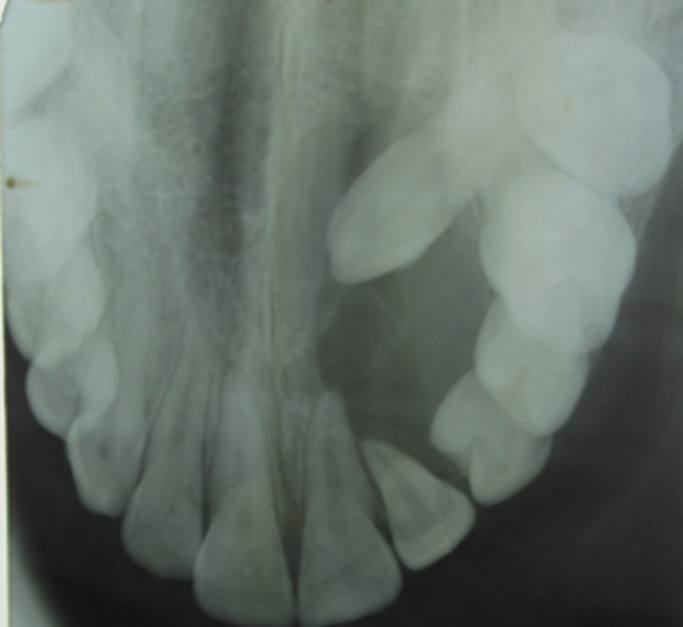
preoperative occlusal radiography
